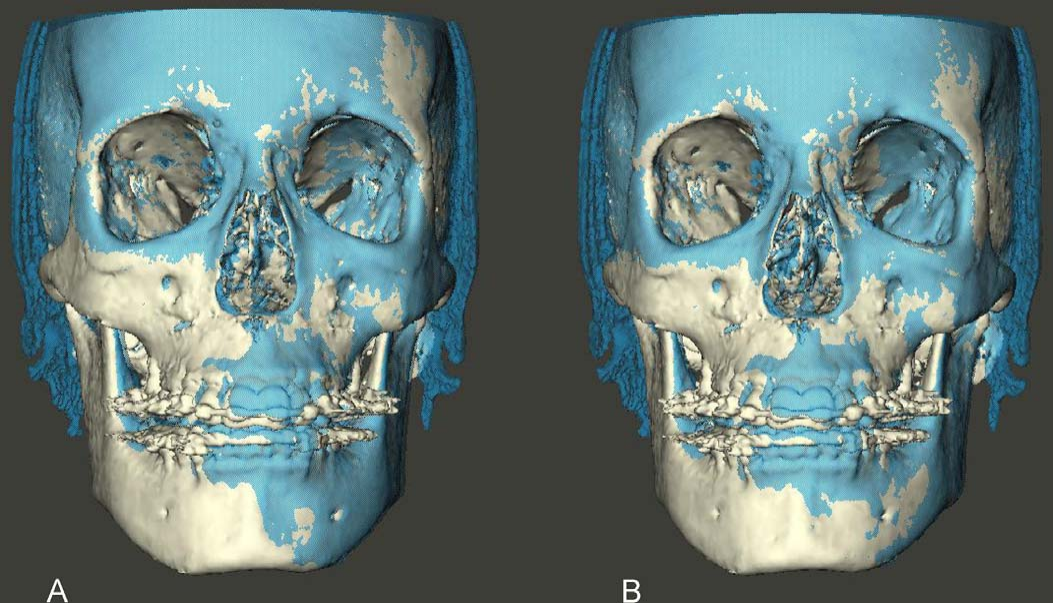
Transparency overlay of superimposed 3D CBCT models. Frontal view. (A) models registered on the anterior cranial base. (B) same models registered on the left zygomatic arch.
